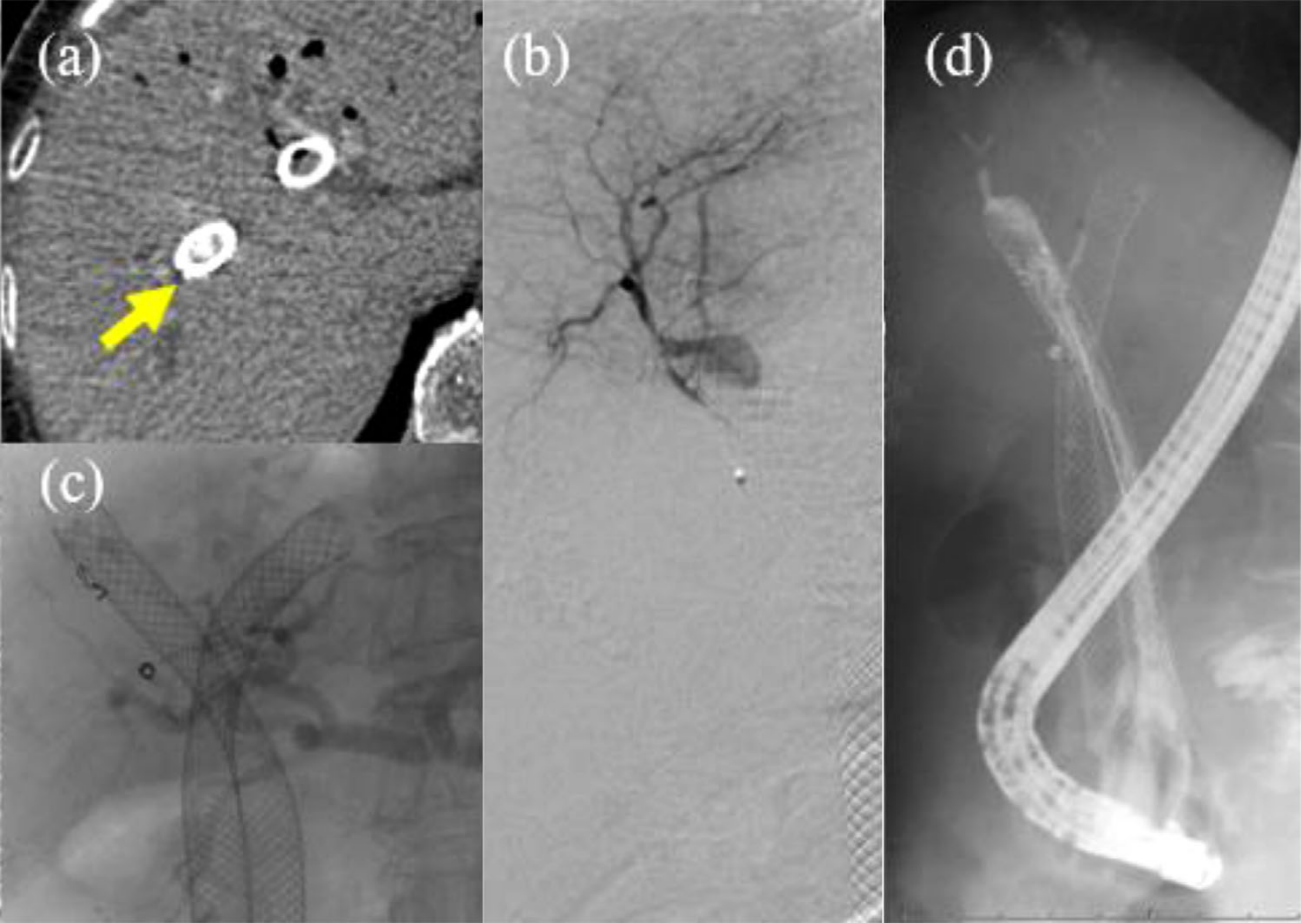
(a) CT scan results showing a pseudoaneurysm in SEMS. The yellow arrow indicates a pseudoaneurysm in SEMS (b) Angiography showing a pseudoaneurysm. (c) Angiography showed A8 of caliber immobility along the upper edge of the stent and pseudoaneurysm in SEMS was noted. Coiling was performed on A8 and the origin of A8 near the pseudoaneurysm. Angiography in the pseudoaneurysm disappeared, but blood flow in A8 remained; therefore, Embosphere was used from the origin of A8. (d) After IVR, ERCP showed a pseudoaneurysm at the upper edge of the right SEMS. CT, computed tomography; SEMS, self‐expandable metal stent. IVR, interventional radiology
